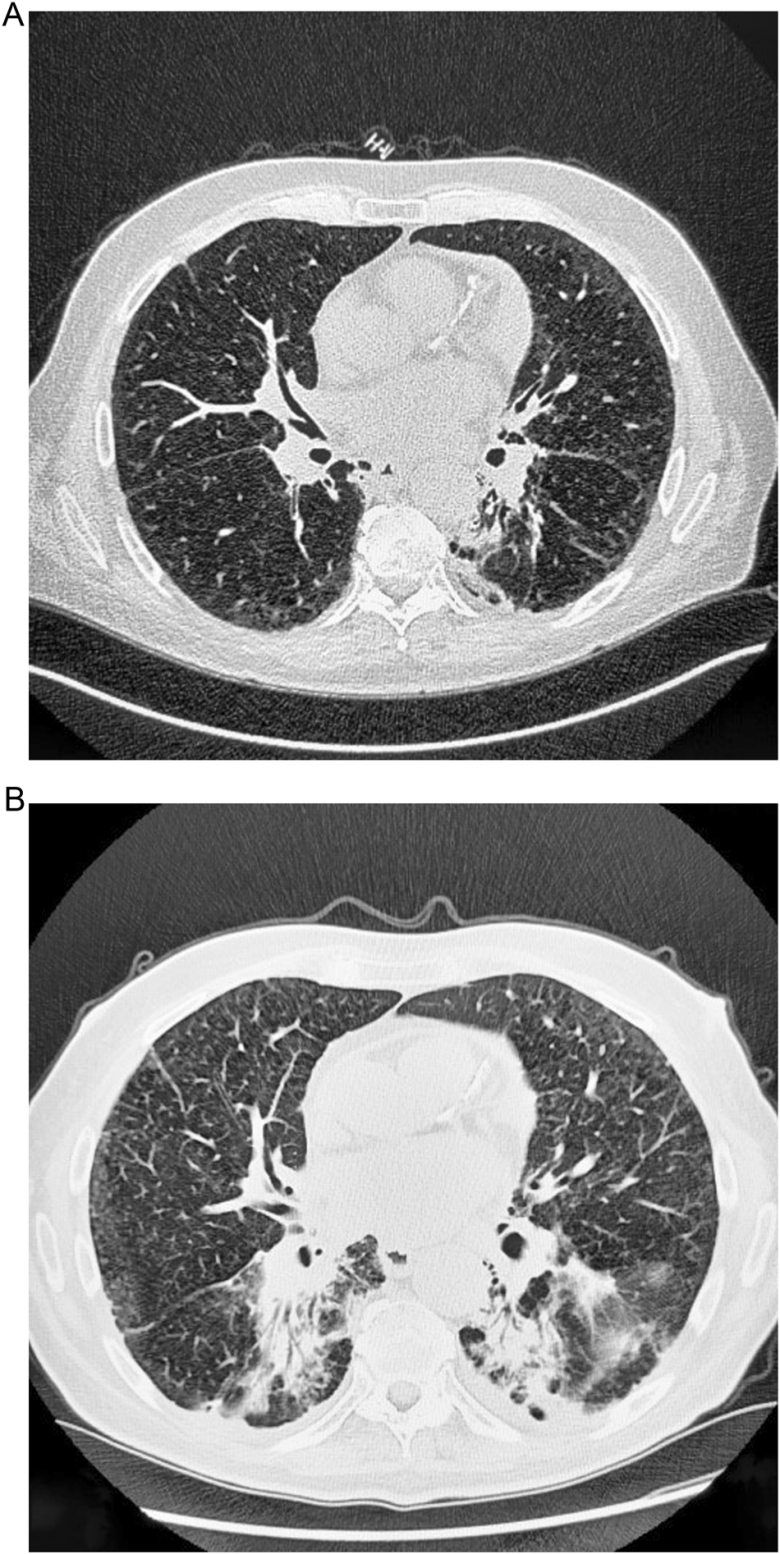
A CT scan of a patient with left-central lung cancer treated with definitive radiochemotherapy. Due to a recurrence 2 years later in the form of mediastinal lymphadenopathy, the patient was treated with monoimmunotherapy and after four cycles the patient developed recall pneumonitis; B the diagnosis of which was established by consensus within a multidisciplinary team. Images reproduced with permission from Dinkel J. 2023. University Hospital LMU Munich, Munich, Germany
